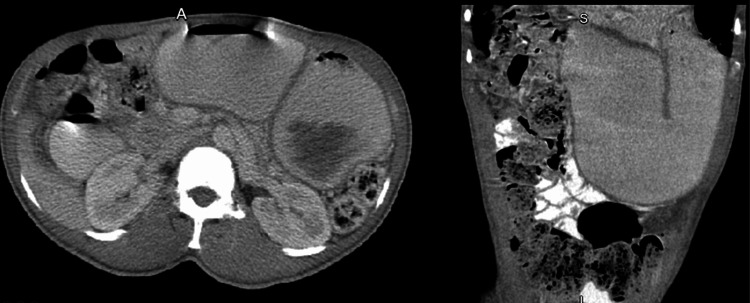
Abdominal CT revealing dilated stomach indicative of gastric outlet obstruction and/or gastroparesis.
